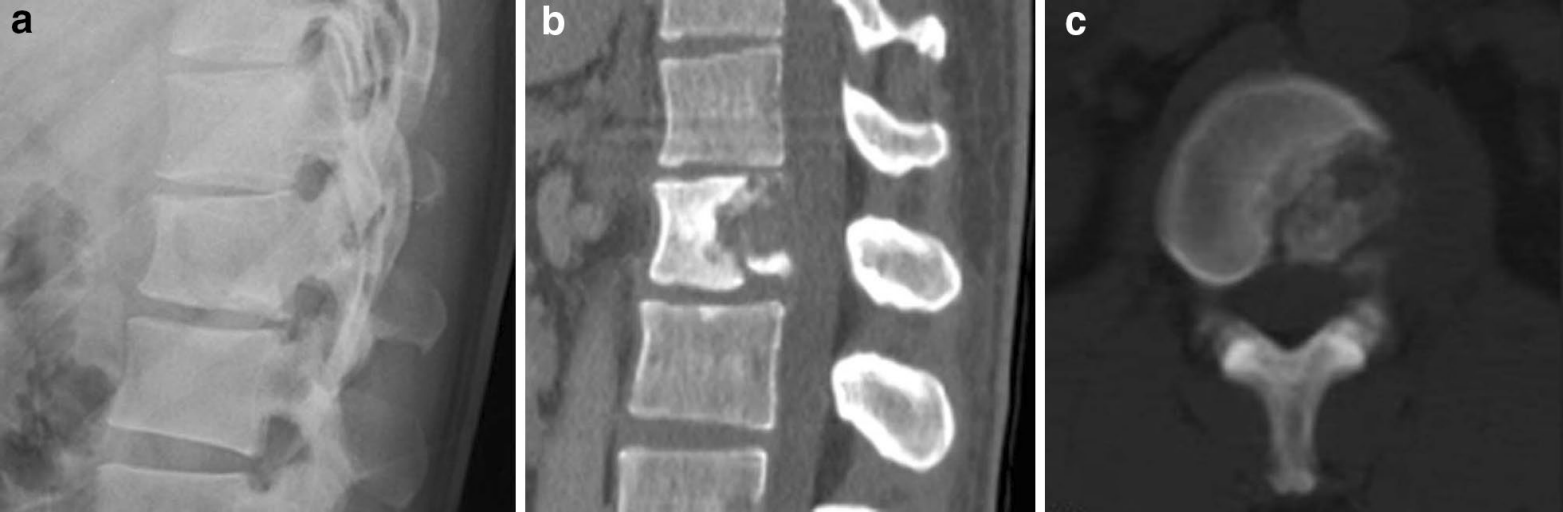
a Plain lumbar X-ray lateral view showing radiolucency of the L1 vertebral body. Sagittal (b) and axial (c) computed tomography showing an osteolytic lesion of the L1 vertebral body
